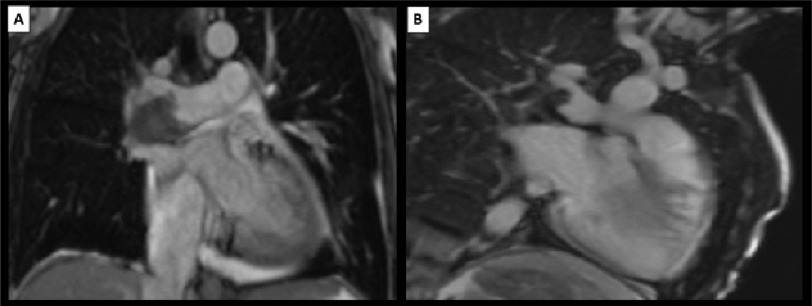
(A) Localizer sequence of cardiac MRI is showing low signal
